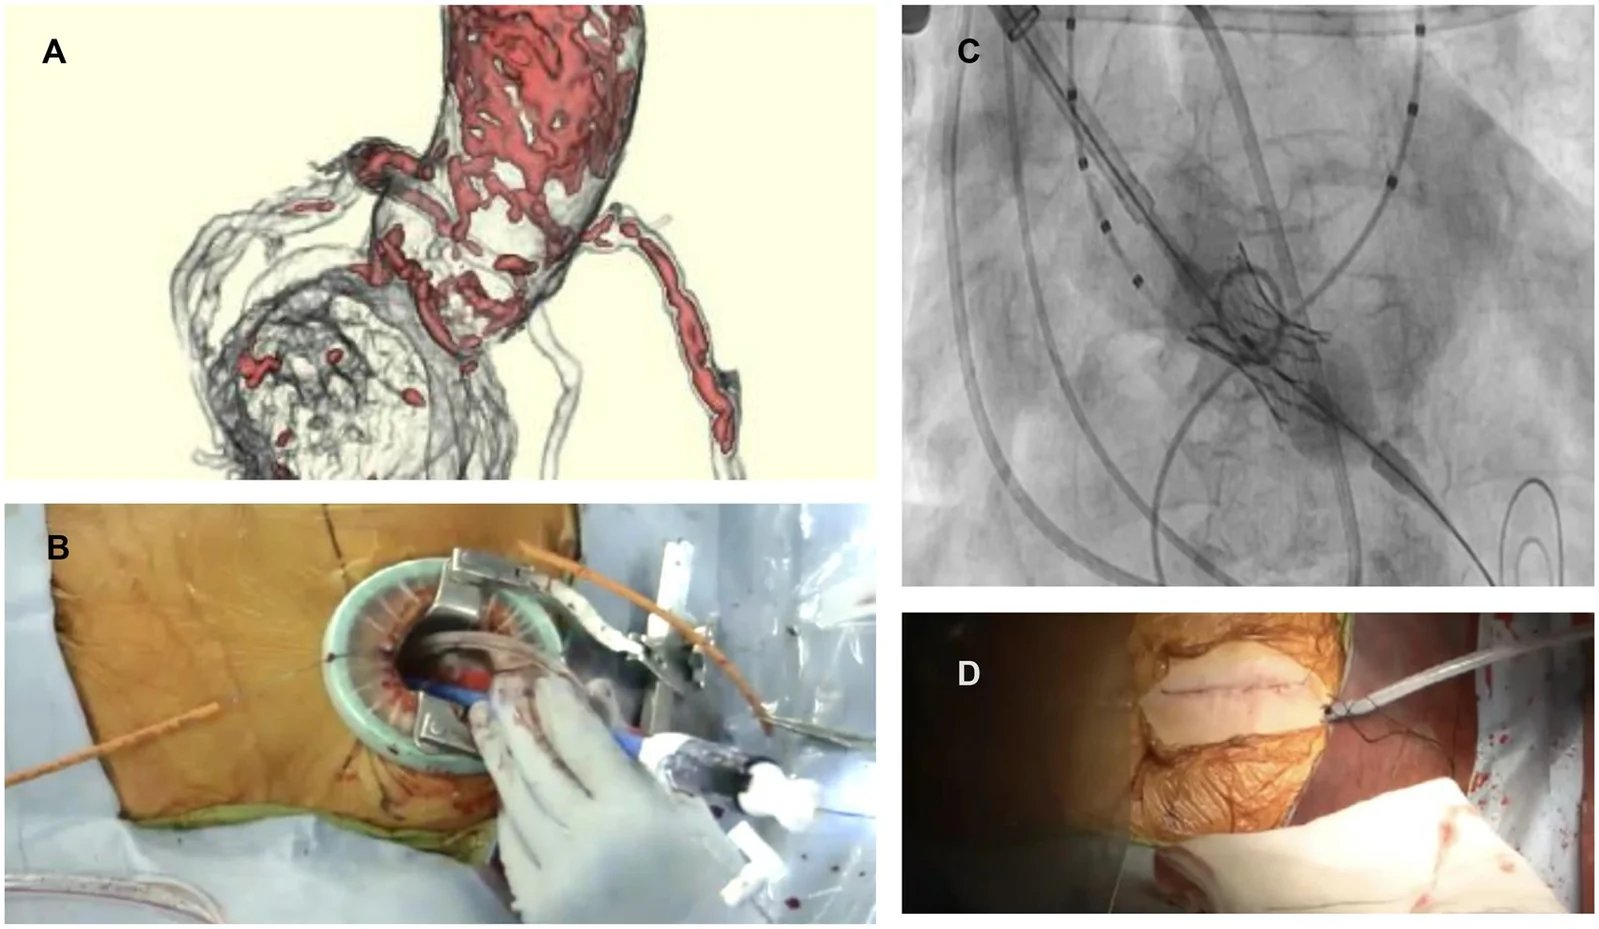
Main Steps of Rt-TAo TAVI. (A) Preprocedural assessment. Circumferential calcification at the intended ascending aortic puncture site was considered a contraindication to the Rt-TAo approach. (B) Manipulation of the ascending aorta. The ascending aorta was exposed through the second or third intercostal space. Delivery sheath was inserted into the ascending aorta. (C) Valve delivery and deployment. The transcatheter heart valve was advanced across the native aortic valve and deployed under fluoroscopic and transoesophageal echocardiographic guidance. (D) Haemostasis and closure. A single pleural chest tube was inserted before wound closure
